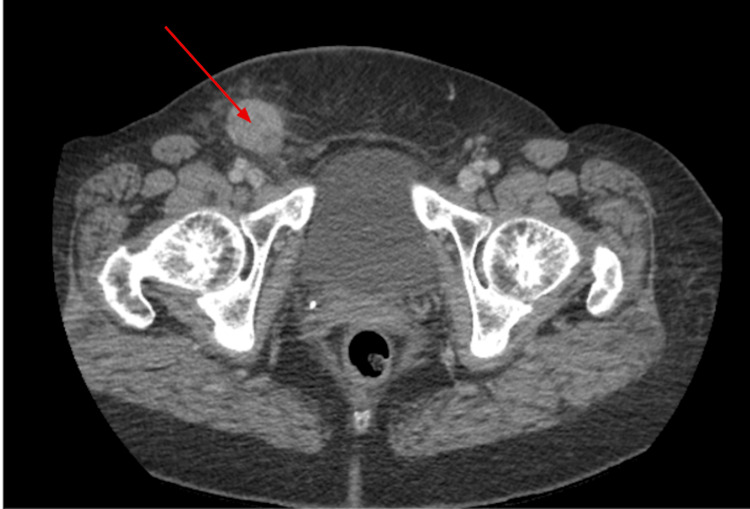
Axial computed tomography of the abdomen and pelvis with contrast demonstrating a right inguinal mass (red arrow). The image demonstrates a pathologically enlarged, enhancing right inguinal and right external iliac/obturator adenopathy, consistent with lymphoma or metastatic disease (red arrow).
